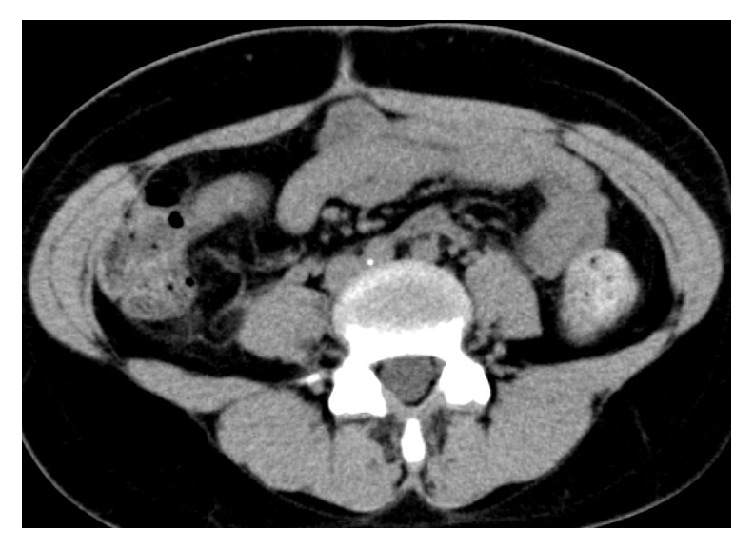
Abdominal plain CT scan one month after surgery. The retroperitoneal mass had disappeared.
